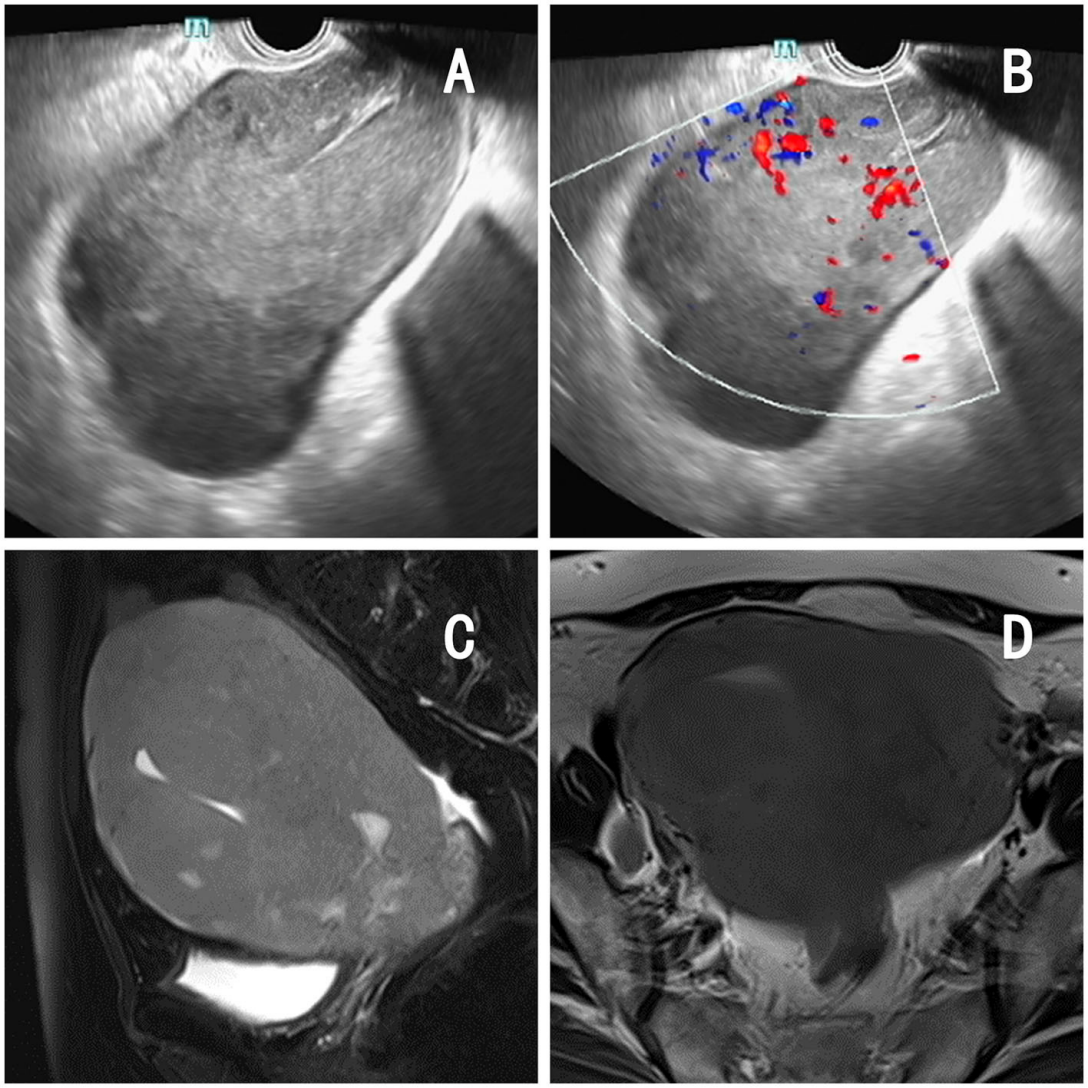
Imaging findings of the patient. (A, B) Pelvic ultrasound: a 13.4 × 9.4 × 8.2 cm mass in the uterine region with clear borders, with rich blood flow signal detectable on CDFI and arterial spectrum detectable. (C, D) Pelvic MRI: significant increase in uterine volume, thickening of the muscular wall, measuring 5.6 cm at the thickest point, accompanied by diffuse abnormal signals and enhancement changes.
